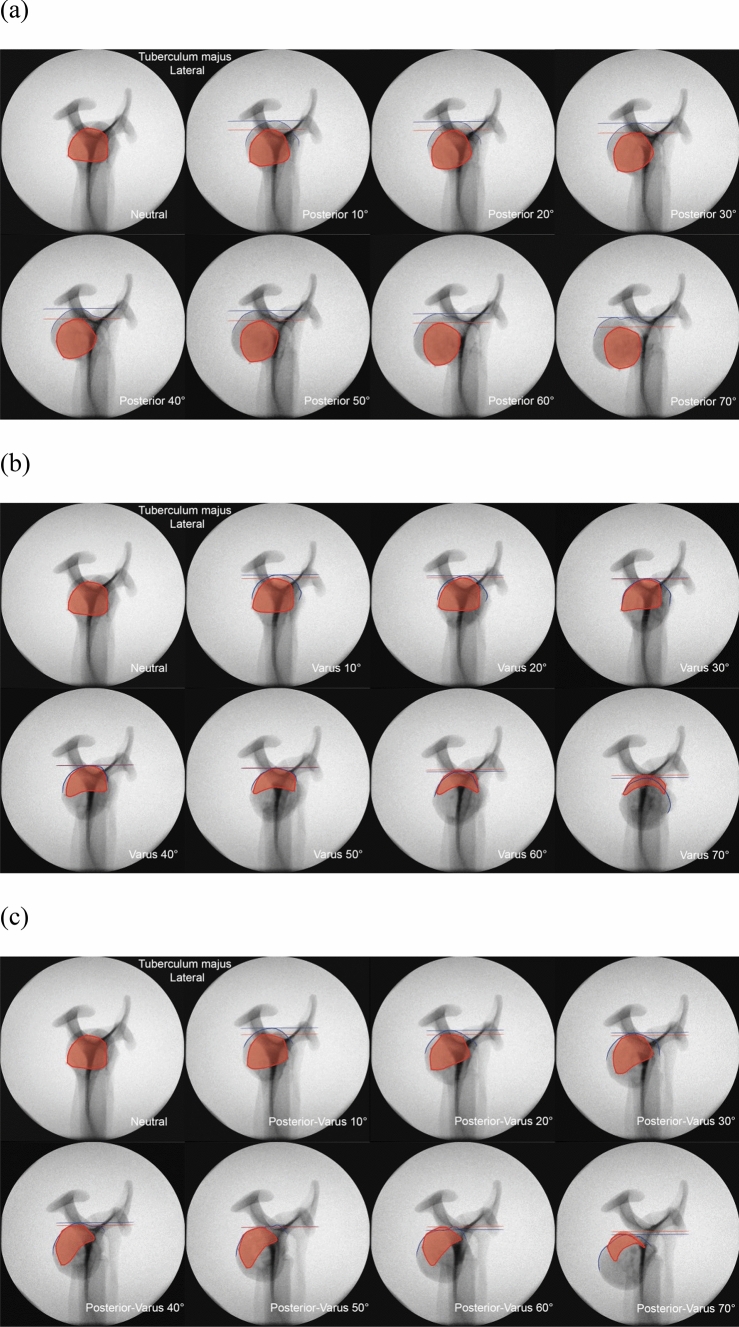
Scapular *Y*-view radiographic series of the greater tuberosity (red) with increasing a posterior, b varus, and c combined posterior-varus angulation. Blue and red lines indicate the most proximal point on the head contour and tuberosity, respectively
